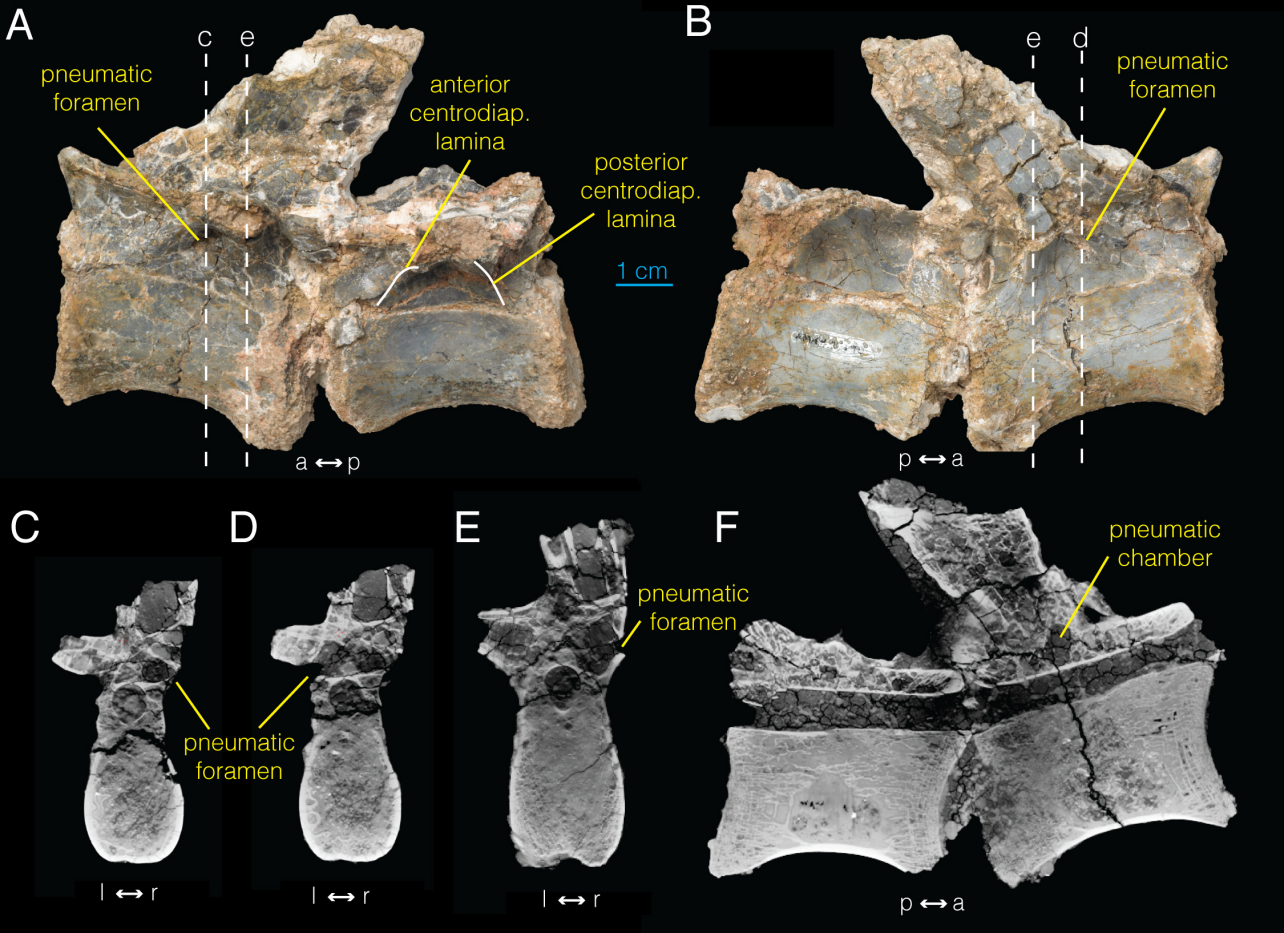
Proximal caudal vertebrae of *Archaeornithomimus* (AMNH FARB 21790) and associated CT images. A, left lateral view; B, right lateral view; C–E, select transverse section; F, midsagittal section. Dashed lines and associated letters indicate location and letter designation of CT image slices.
